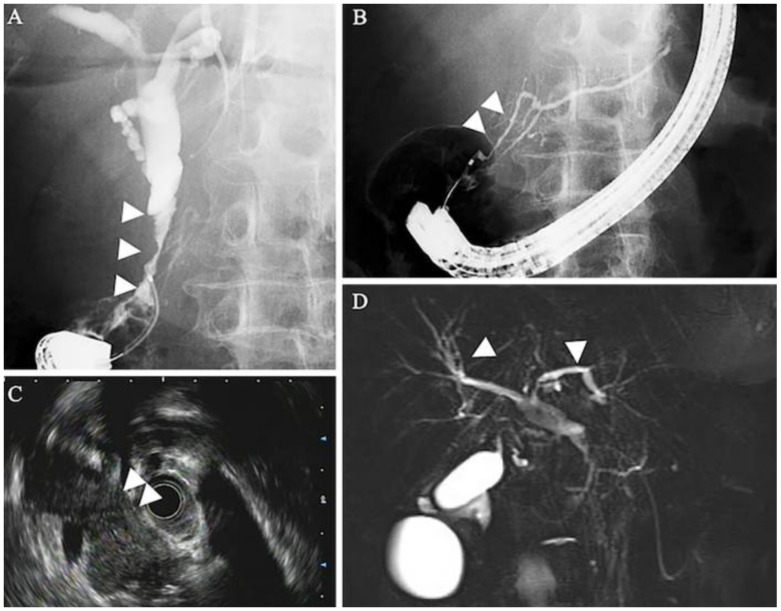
Imaging findings from endoscopic retrograde cholangiopancreatography (ERCP), endoscopic ultrasound (EUS), and magnetic resonance cholangiopancreatography (MRCP) in a patient with AIP-3. (A) Endoscopic retrograde cholangiography demonstrated stenosis of the intrapancreatic bile duct (arrowheads). (B) Endoscopic retrograde pancreatography revealed narrowing of the main pancreatic duct at the head of the pancreas (arrowheads). (C) EUS identified a diffuse hypoechoic enlarged pancreas (arrowheads). (D) MRCP showed no evidence of intrahepatic bile duct wall thickening or stenosis [54].
